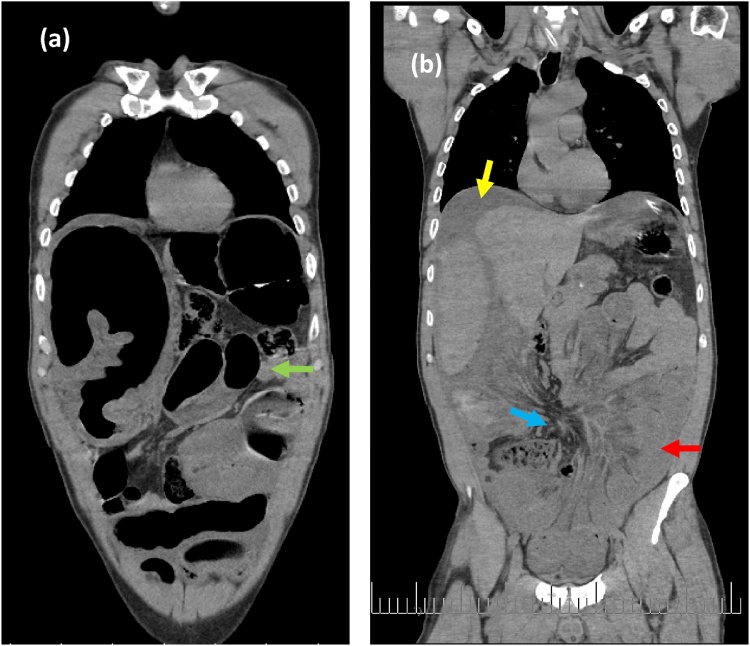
Abdominal CT with contrast, two coronal views: (a) white arrow shows suspected sigmoid volvulus in the right upper quadrant. Green arrow shows dilated small bowel loop. (b) yellow arrow demonstrates preihepatic free fluid, red arrow points at non-enhancing small bowel loop and edematous roots of mesentery, blue arrow shows swirl sign and the suspected site of hernia aperture.
